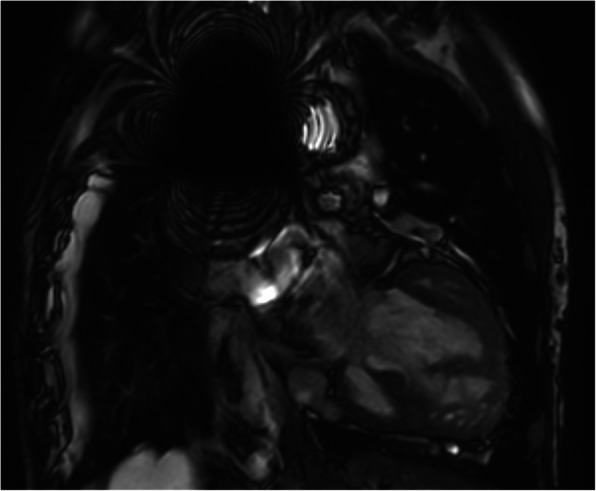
Sagittal steady-state free precession MRI image of the superior mediastinum. A large metal artifact caused by a surgical clip obscures the image and renders flow evaluation impossible
